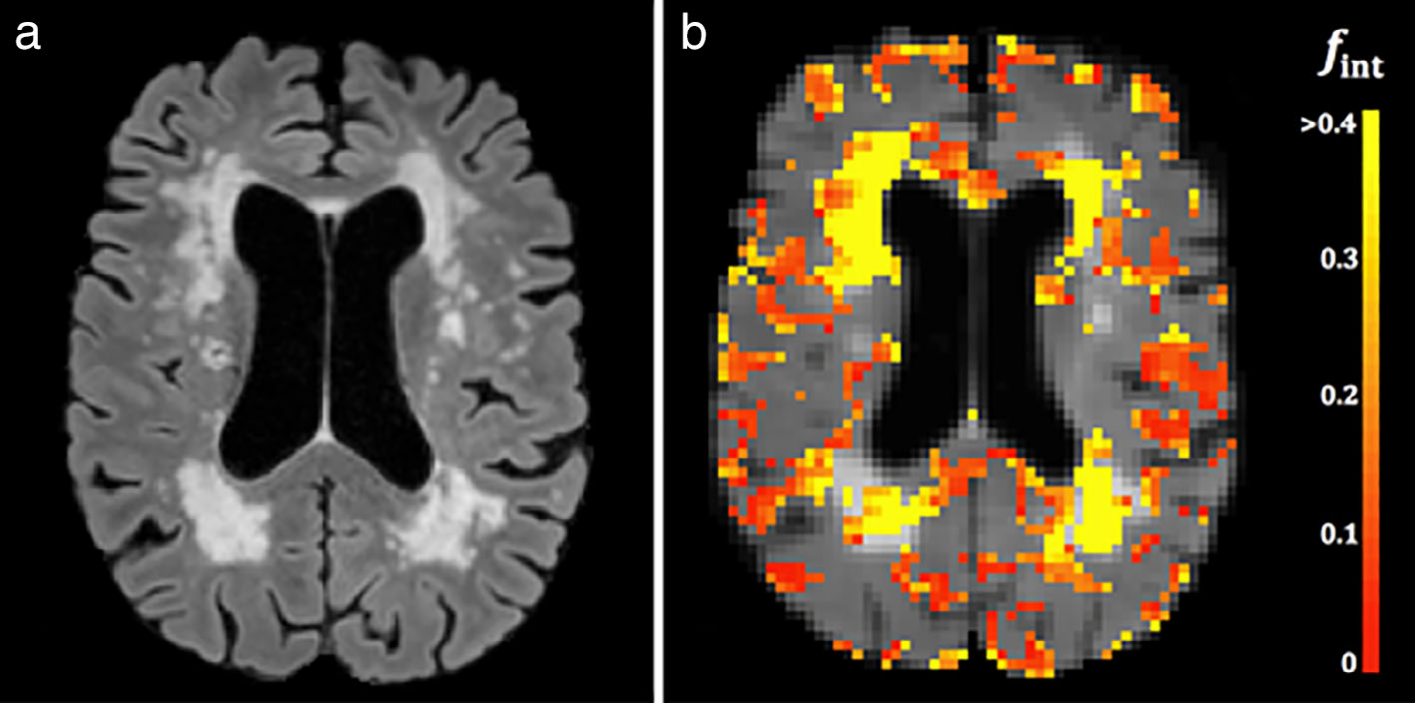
FLAIR image (a) and superimposed color‐coded map of intermediate diffusion volume fractions (b) of a patient (male, 79 years) with cerebral small vessel disease. High values of intermediate diffusion volume fractions strongly colocalize with white matter hyperintensities. Please note that pericortal regions with elevated intermediate diffusion volume fractions are likely due to partial volume effects. For display purposes, the CSF in the ventricle has been masked out.
